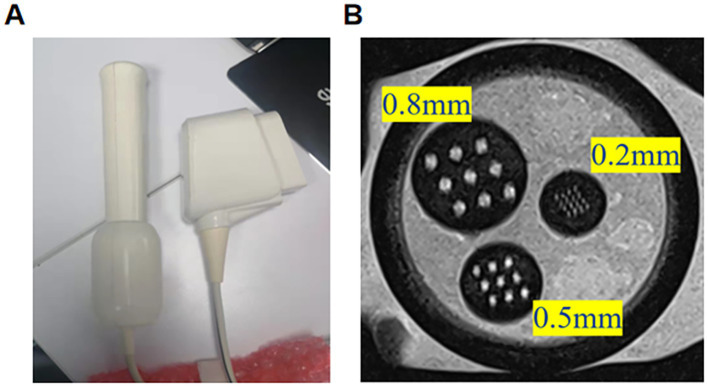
(A) Appearance of the vaginal cavity coil. The vaginal insertion portion is on the right, and the connector is on the left. (B) In vitro standard model imaging at 3.0T MRI using the vaginal cavity coil. A 0.2 mm diameter hole in the model is clearly visualized.
